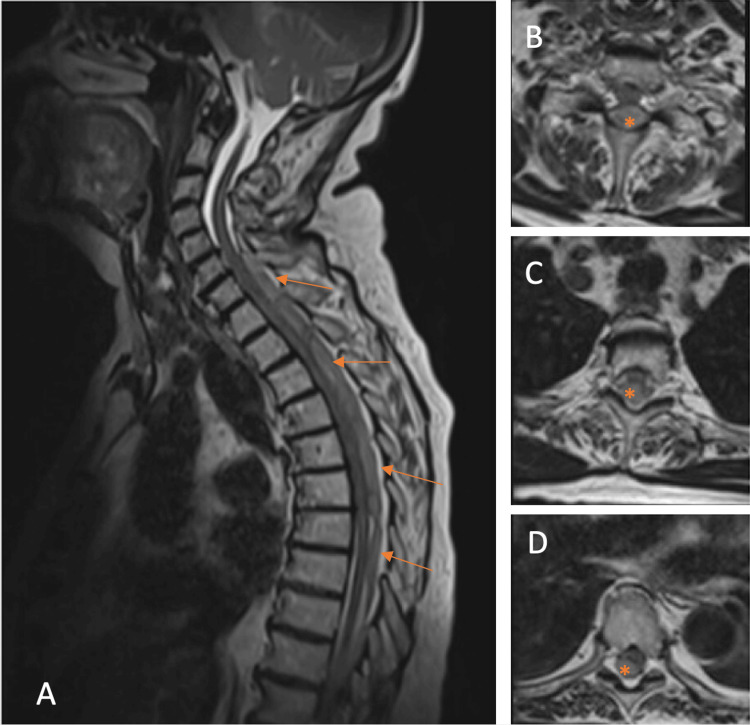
Magnetic resonance imaging (MRI) of the first case at presentation, demonstrating an epidural spinal hematoma (arrows and asterisks) extending from C5 to T8. (A) Sagittal T2-weighted image; (B) axial T2-weighted image at C7; (C) axial T2-weighted image at T3; (D) axial T2-weighted image at T6.
